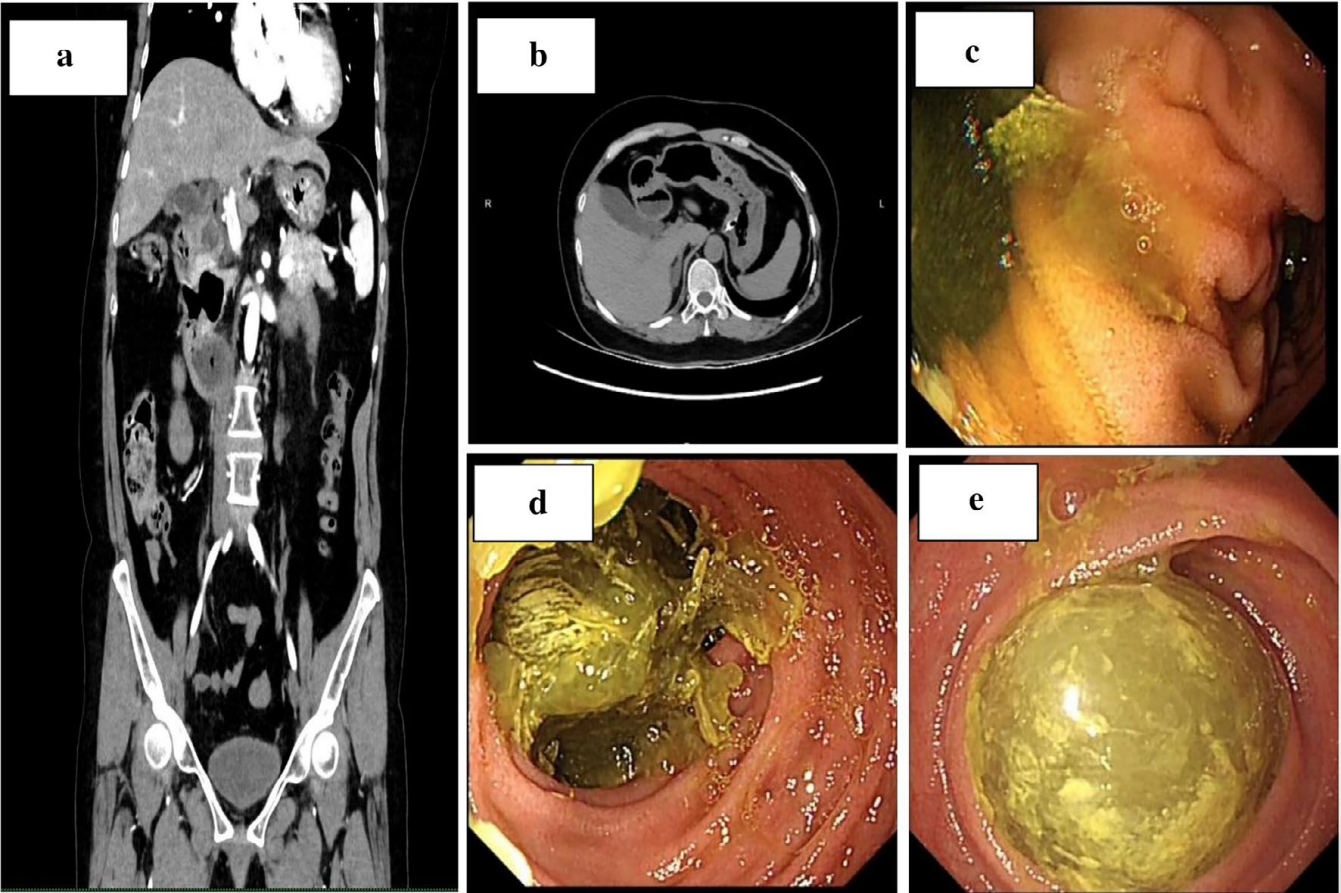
(a) Contrast‐enhanced computed tomography. Duodenal bulbar dilatation with diverticulum (coronal view). (b) Contrast‐enhanced computed tomography. Duodenal bulbar dilatation with diverticulum (axial view). (c) Gastroscopy. Duodenal diverticulum. (d) Gastroscopy. Duodenal obstruction. (e) Single‐balloon enteroscopy. A 2.5‐cm globular enterolith 280 cm from the pylorus.
